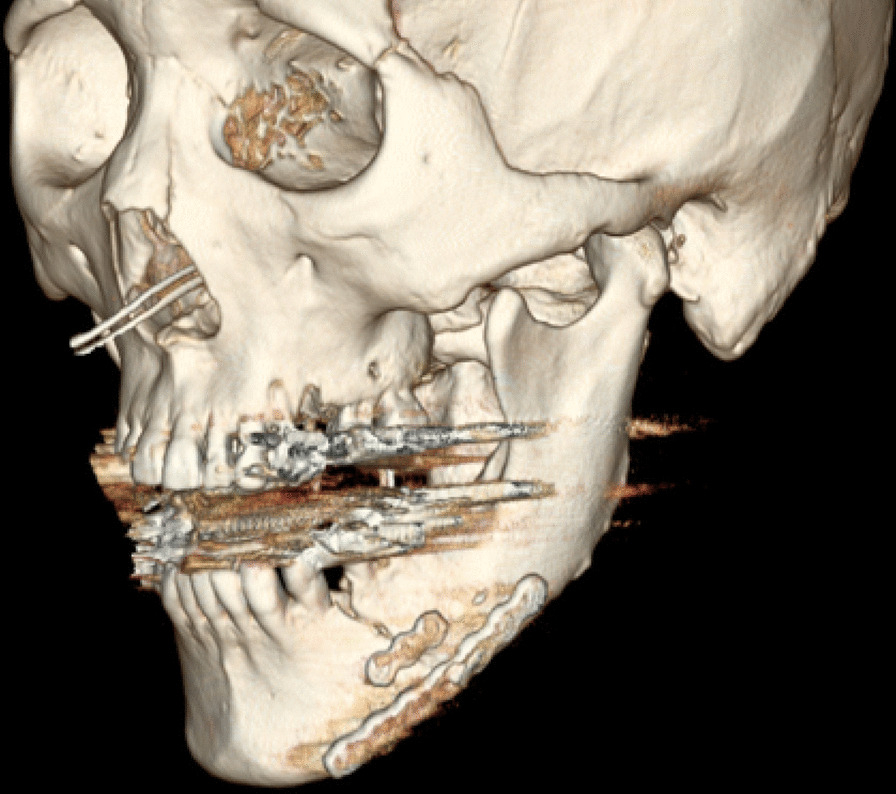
Three-dimensional computed tomographic findings obtained after ORIF showing that adequate reduction and fixation were obtained
